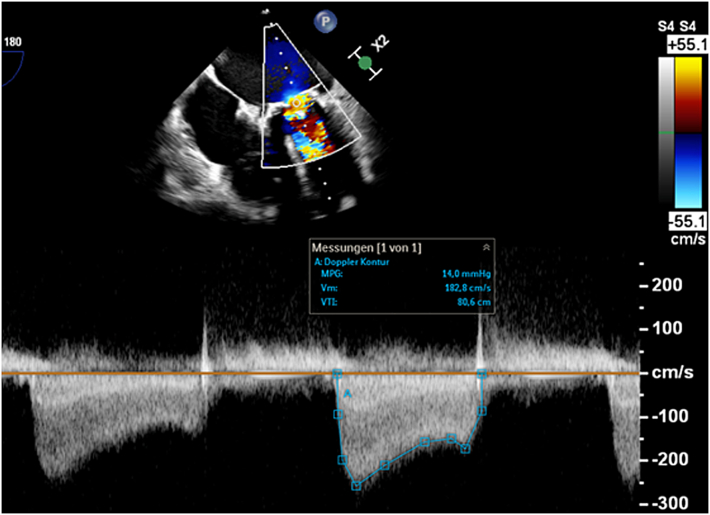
Preintervention Transesophageal Echocardiography Transesophageal echocardiography demonstrating severe stenosis of the bioprosthetic mitral valve; continuous-wave Doppler shows a mean transmitral pressure gradient of 14 mm Hg.
